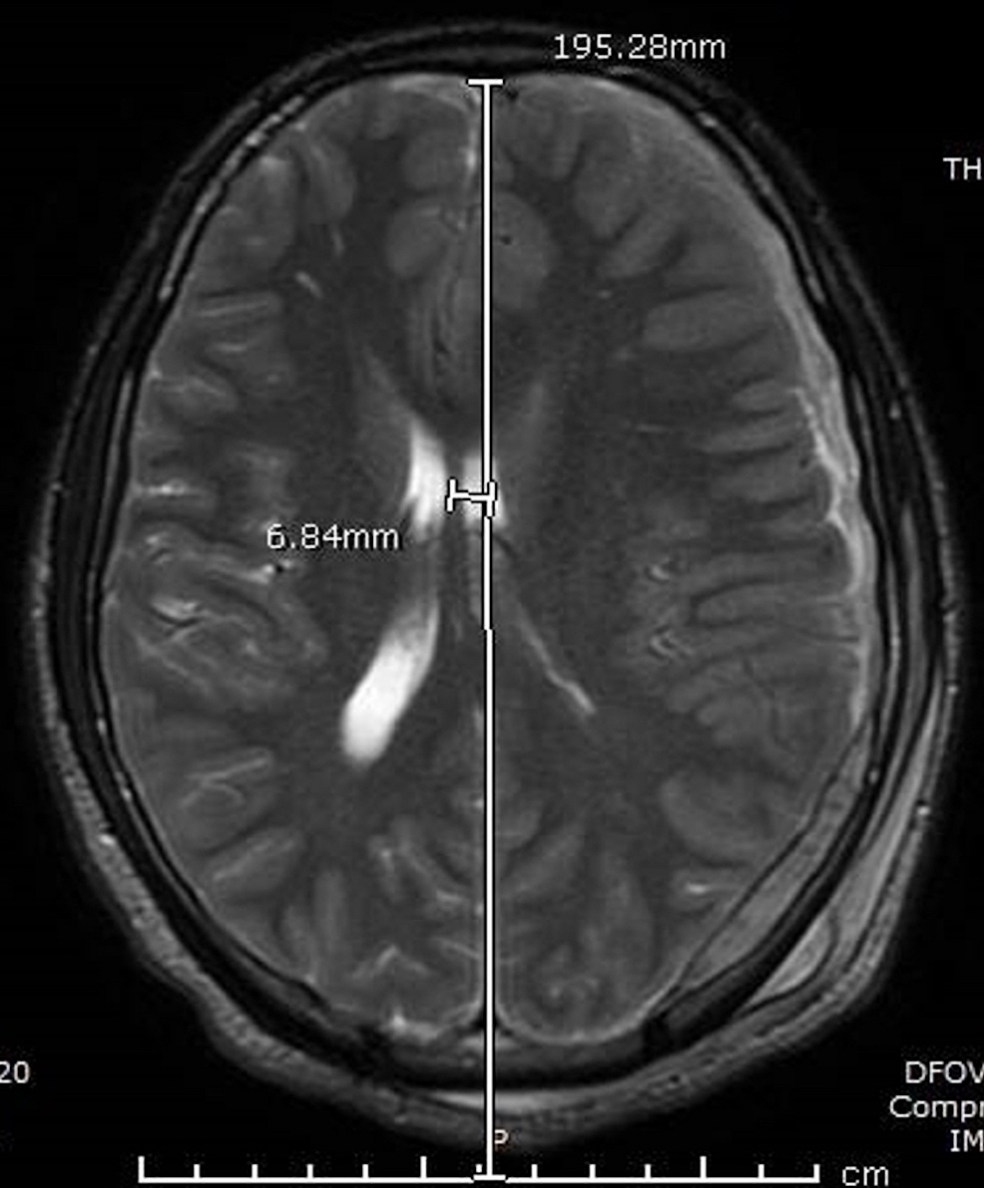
MRI brain axial T2-weighted. The presence of cerebral edema with a 6 mm left-to-right midline shift was evident.
